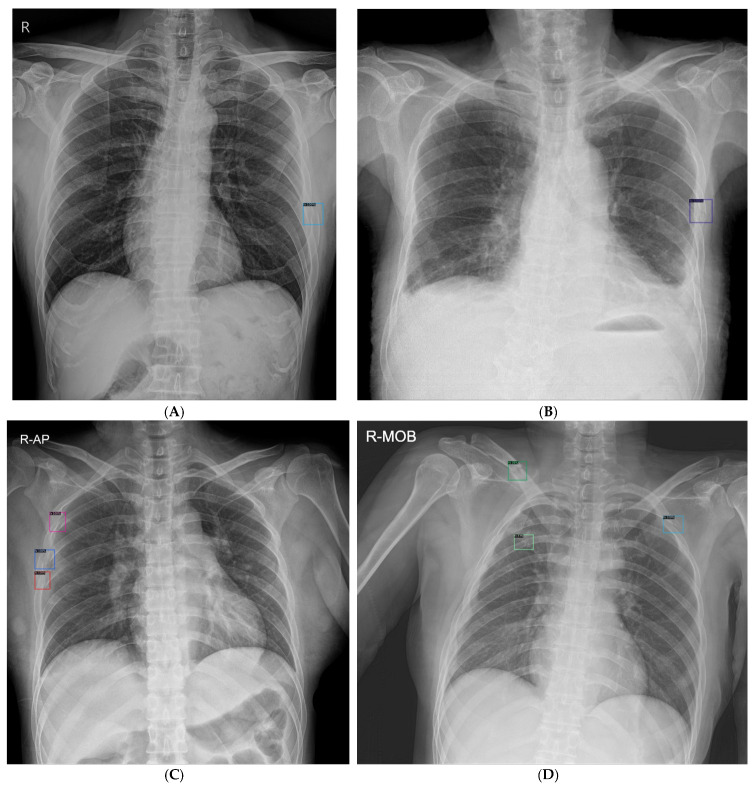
Examples of rib fractures detected on chest radiographs using the AI model (A) Single rib fracture: The AI model’s correct identification of a rib fracture is demonstrated on the left lateral side, marked with a predicted box and assigned a 100% probability of fracture. (B) Single rib fracture in low resolution shows the AI model’s capacity to precisely mark a rib fracture location on a low-resolution image. (C) Multiple rib fractures: This image displays the AI model’s marking of several rib fracture sites, with each predicted box accompanied by a respective estimated probability of fracture. (D) Accurate and erroneous marking: This image features the AI model’s accurate marking of a rib fracture on the left side and a false-positive marking on the right side. Additionally, it includes an unintended marking of a clavicle fracture, not originally part of the training data. AI: Artificial Intelligence.
